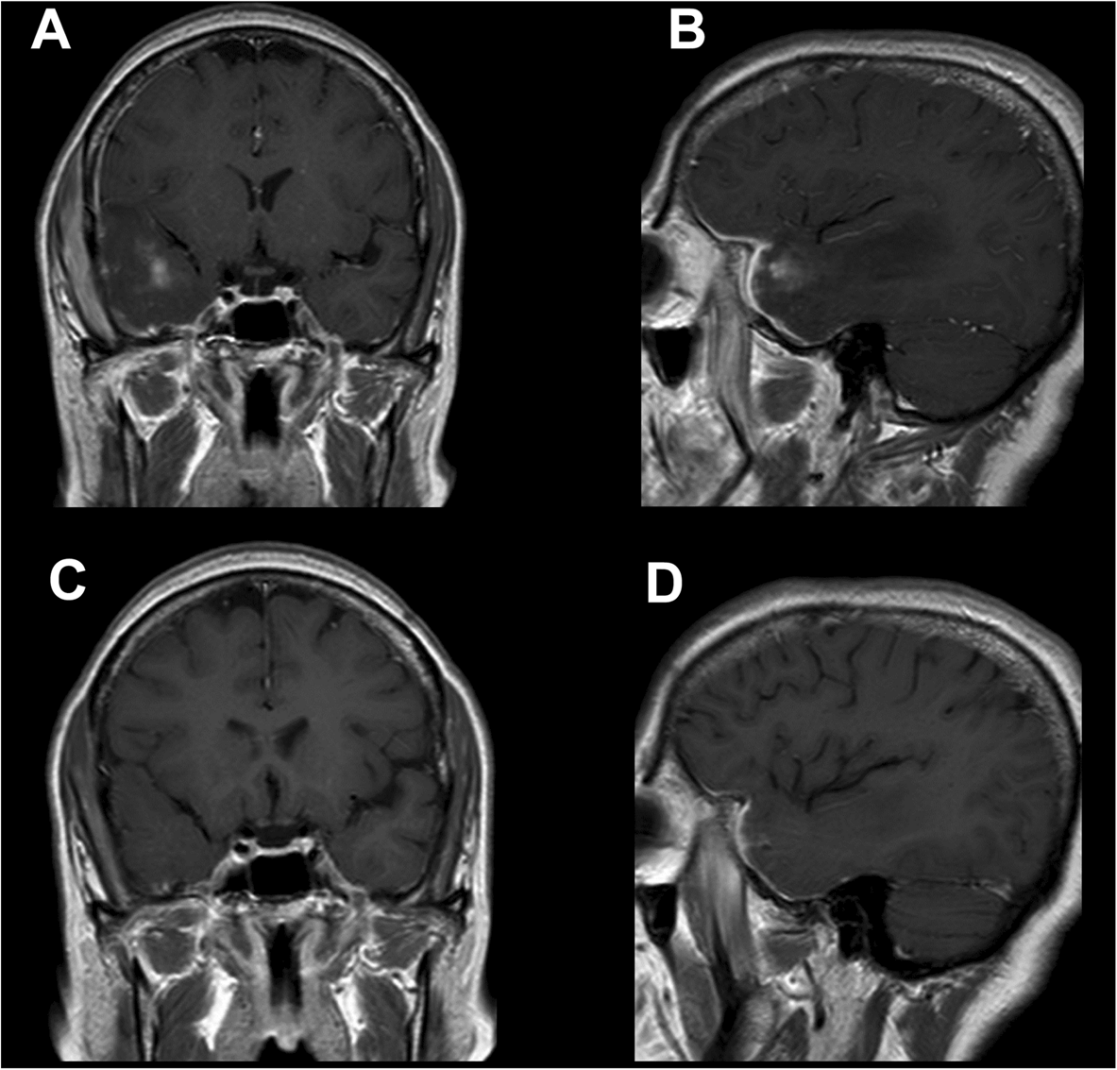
Intracranial enhancement before and after steroid therapy. Coronal (a, c) and sagittal (b, d) CE T1w MR images before (upper row) and after (lower row) steroid therapy. In the follow-up phase, CE-MRI (C-D) shows striking improvement of the dural thickening and complete regression of the intra-axial enhancement
